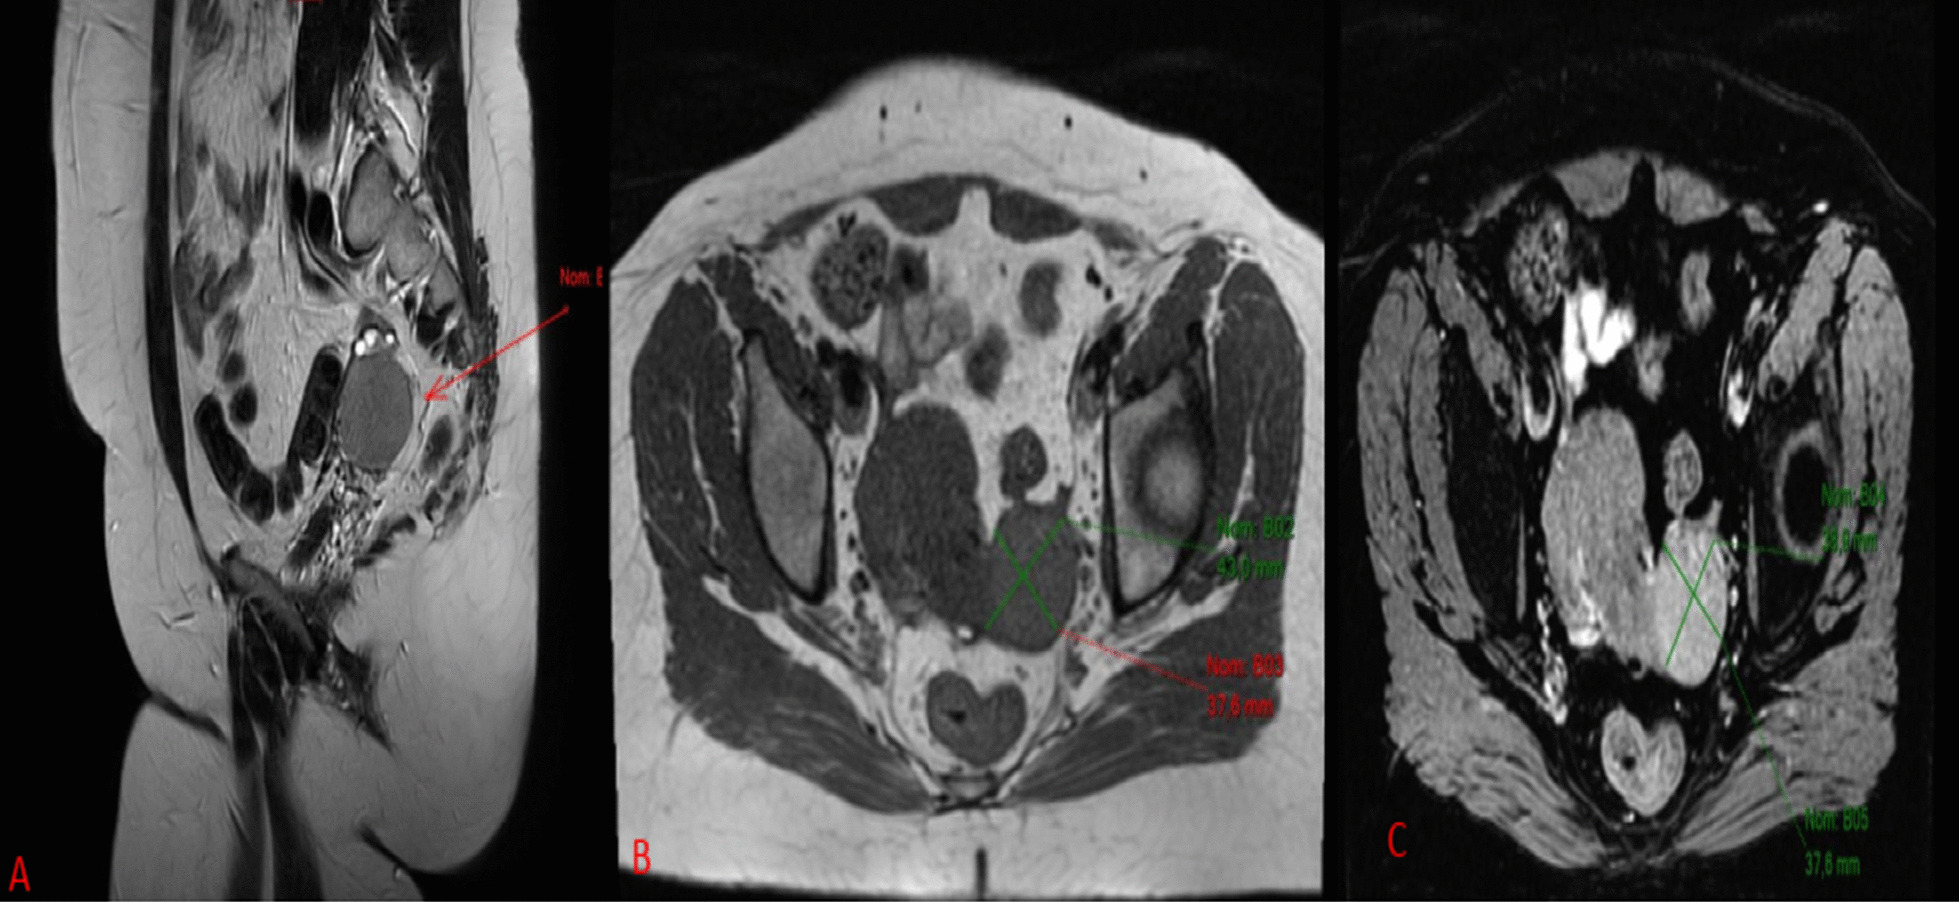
Pelvic MRI showing the presence of a left ovarian mass. A Sagittal slice in T1 sequence, B axial slice in T1 sequence, C axial slice after injection of gadolinium
